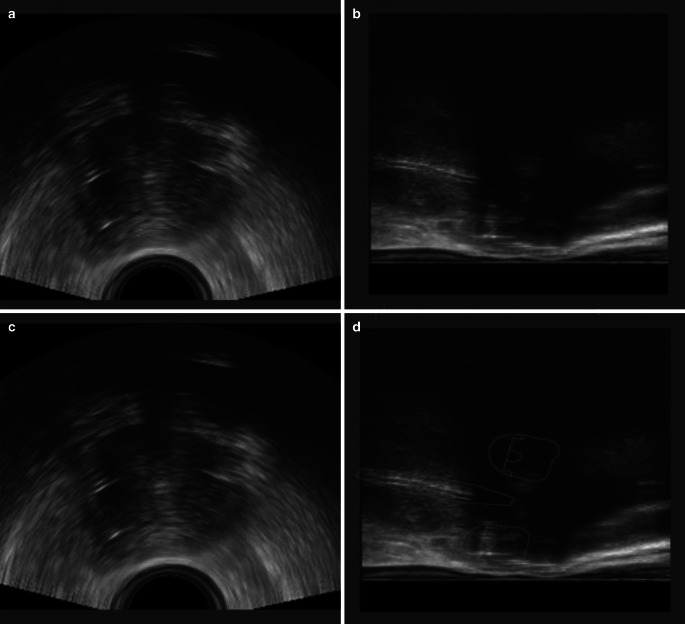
Example transrectal ultrasound (TRUS) images of a patient with FIGO IIB cervical carcinoma at time of brachytherapy with the applicator in place: a axial view; b sagittal view; c axial view with reconstructed applicator; d sagittal view with reconstructed applicator
